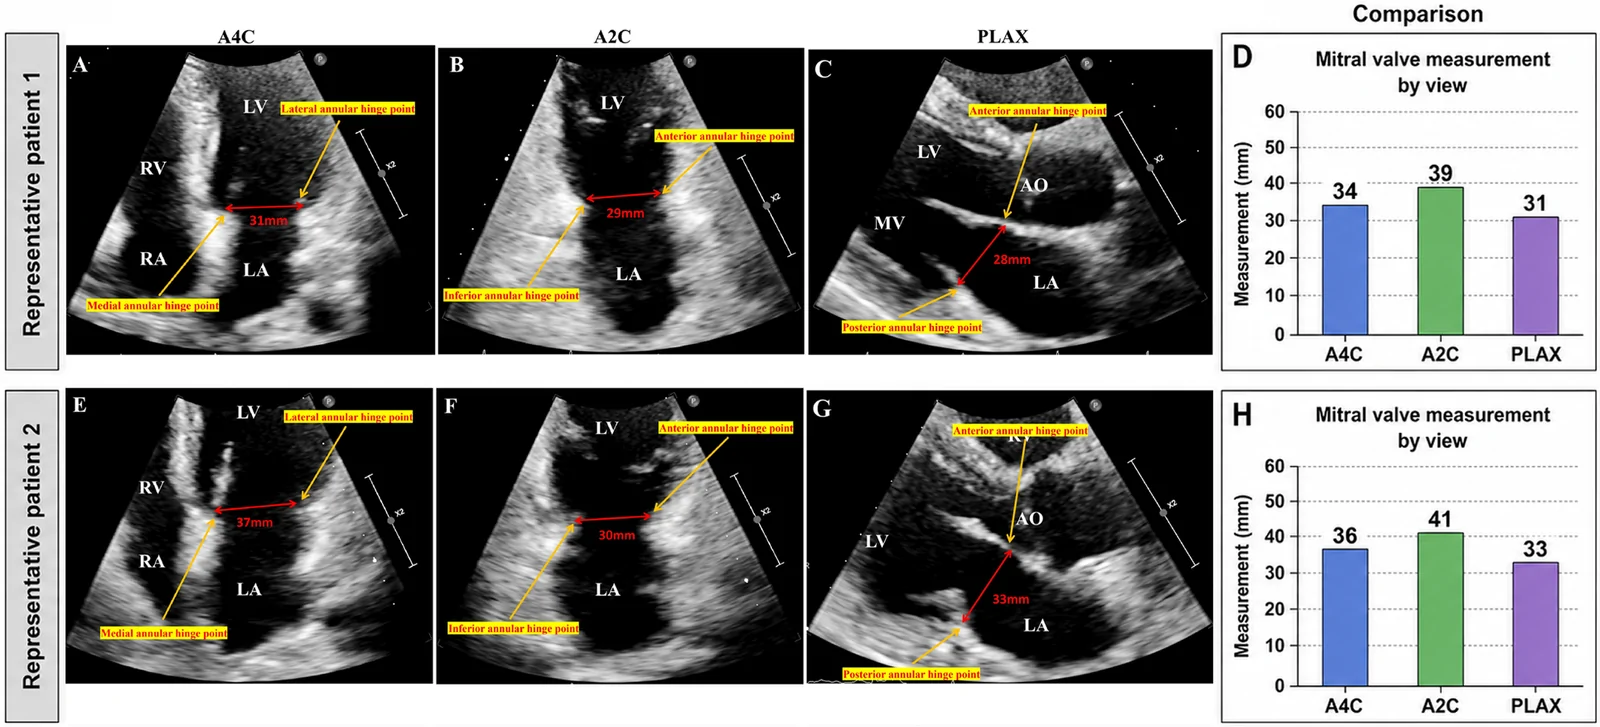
Representative inter-view variability in mitral annular diameter measurements. Mitral annular diameters were measured in the apical four-chamber (A4C), apical two-chamber (A2C), and parasternal long-axis (PLAX) views in two representative patients. Panels A–C and E–G show the corresponding measurements, whereas panels D and H summarize the measurements graphically. Blue calipers indicate the straight-line distance between opposing mitral annular hinge points.
